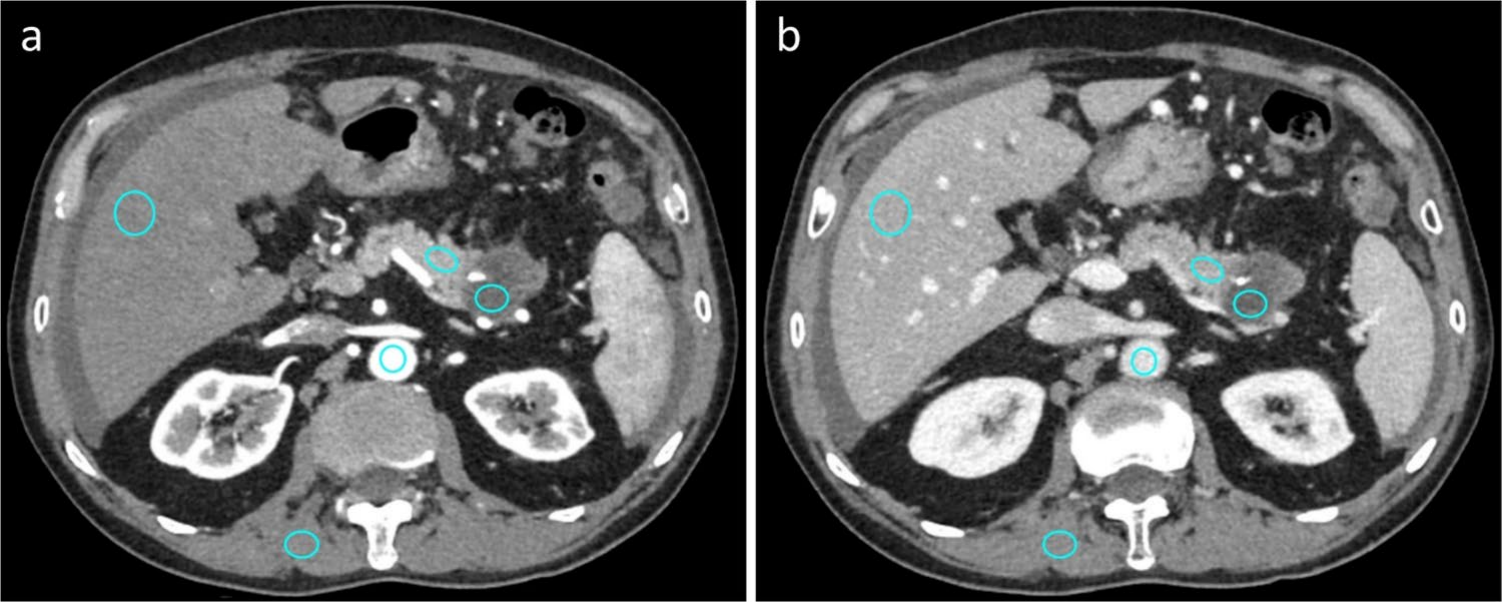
Virtual monochromatic images at 70 kiloelectron-volt (keV) reconstructed using a deep-learning image reconstruction algorithm in the (a) pancreatic and (b) portal venous phase images. To measure CT values, regions of interest were manually drawn on the paraspinal muscle, abdominal aorta, portal vein, liver, pancreas, and pancreatic ductal adenocarcinoma
